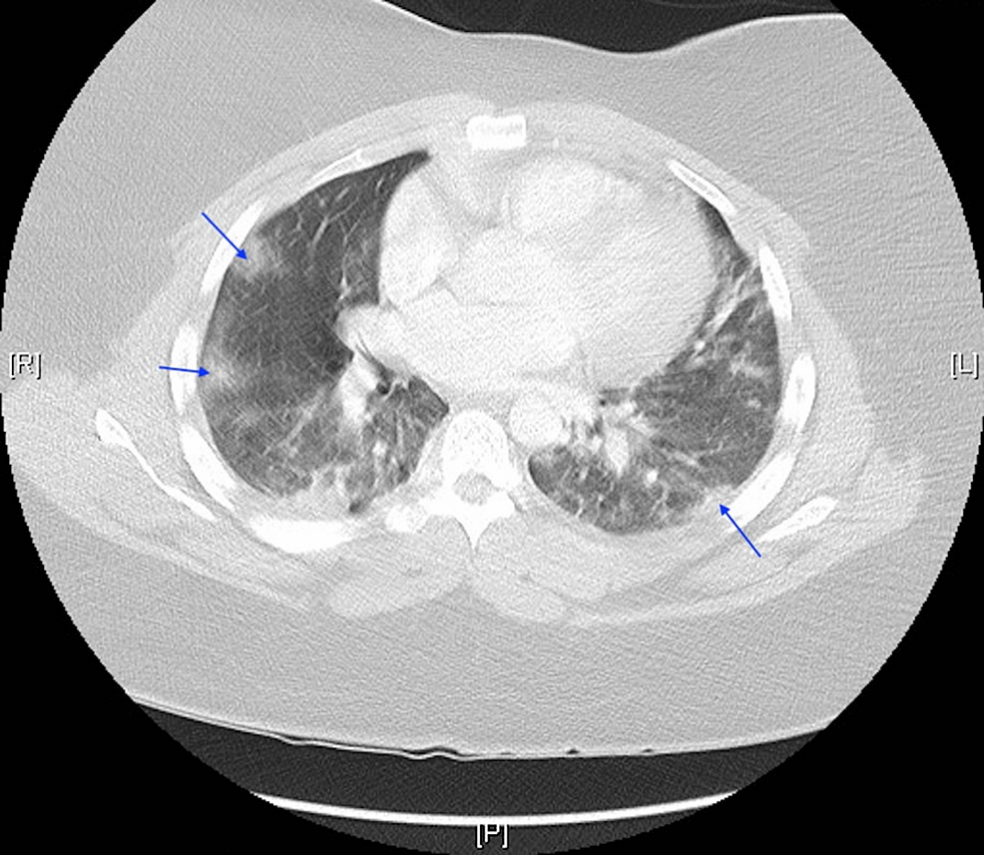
Chest CT demonstrating the classic ground glass opacities seen with COVID-19 infection
